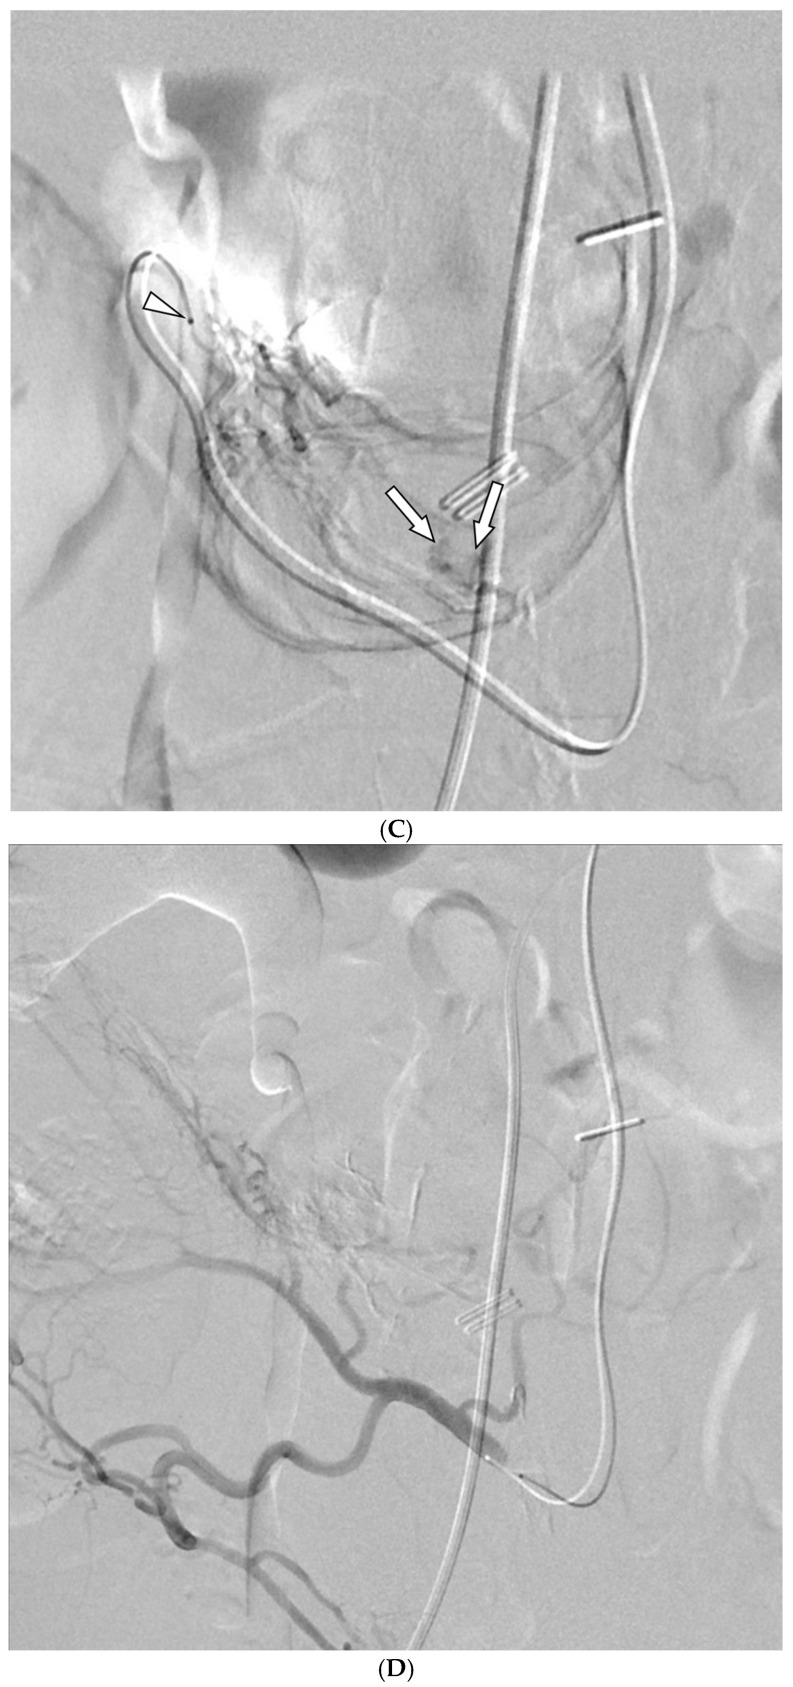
A 78-year-old man presented with hematochezia. (A) Contrast-enhanced axial CT image shows luminal contrast extravasation (arrow) at the ileal loop. (B,C) Selective ileal arteriography shows contrast extravasation (arrows). Due to the distance and tortuosity of the path from the microcatheter tip (arrowheads) to the bleeding focus, superselection was not possible; therefore, embolization was performed using quick-soluble gelatin sponge particles (350–560 µm). (D) Post-embolization arteriography demonstrates that the bleeding focus and the arterial branches leading to it are no longer visible. No further bleeding occurred during the 1-year follow-up.
